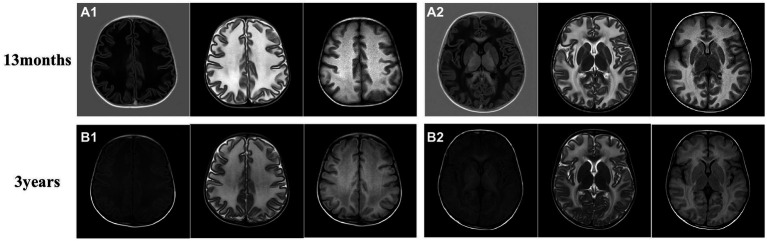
Brain magnetic resonance imaging (MRI) of the proband. (A) The brain MRI of the proband at 13 months. (B) Another image was taken at 3 years old. The MRI revealed swelling in the bilateral cerebral cortex and diffuse signal abnormalities in cerebral white matter, showing a low signal on T1-weighted images and a high signal on T2-weighted images, as well as in fluid-attenuated inversion recovery images. Subcortical cysts were not observed in any section.
